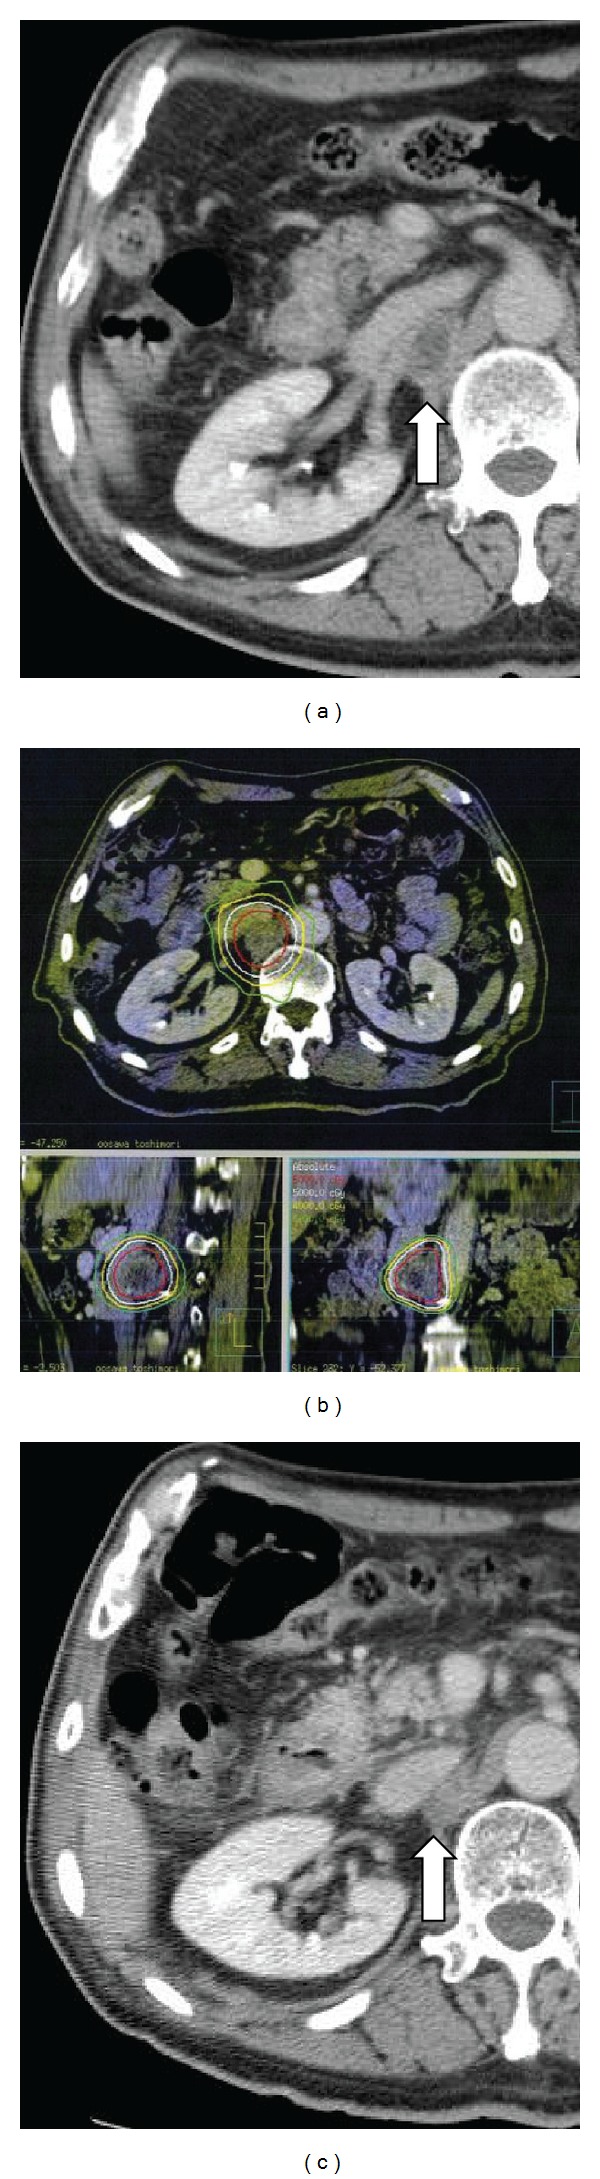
(a) CT of right para-aortic lymph node metastasis (arrow) before SBRT. (b) Dose distribution made with 10 noncoplanar beams for SBRT. Isodose lines show total doses (in 20 fractions) of 60 Gy, 50 Gy, 40 Gy, and 30 Gy, respectively, from the innermost area. (c) CT at 4 months after SBRT, showing partial response of the lesion (arrow).
